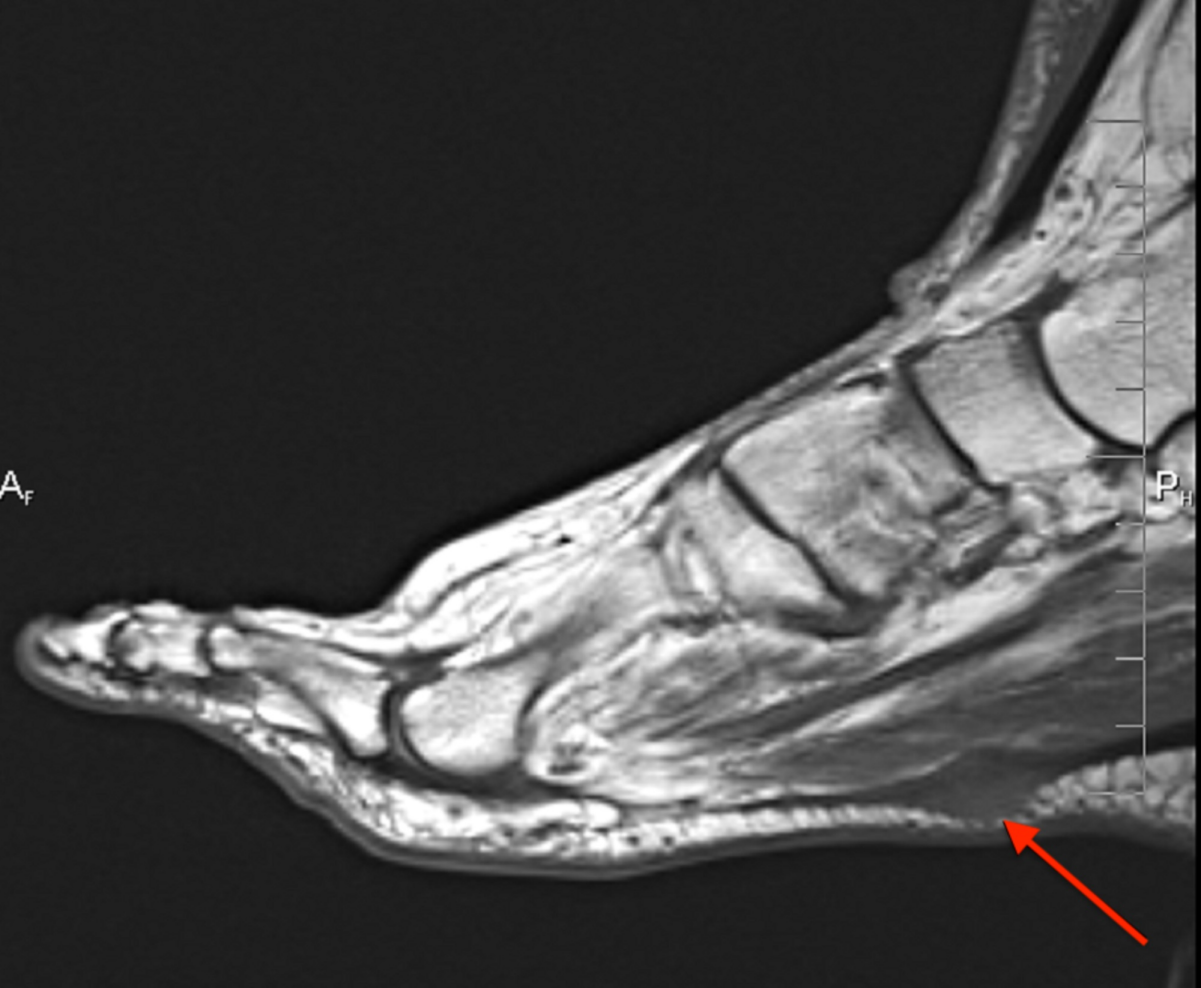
Case 3 MRI showing the T1 weighted sagittal image of the right foot demonstrating a plantar fibroma within the central portion of the plantar cord.
